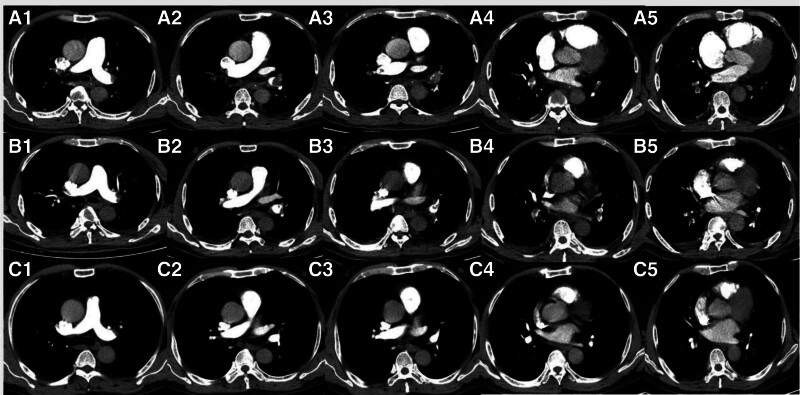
(A1–A5) One week after acute cerebral infarction, computed tomographic pulmonary angiography showed the left and right pulmonary artery trunks and grades II–III major branch emboli. (B1–B5) Five days after pulmonary artery thrombectomy, CTPA showed emboli in the trunks and main branches of the left and right pulmonary arteries. These were significantly absorbed compared with the initial stage of the disease. (C1–C5) Three months after discharge, a CTPA reexamination showed that there are no emboli in the main pulmonary arteries or their branches. CTPA = computed tomography pulmonary angiography.
